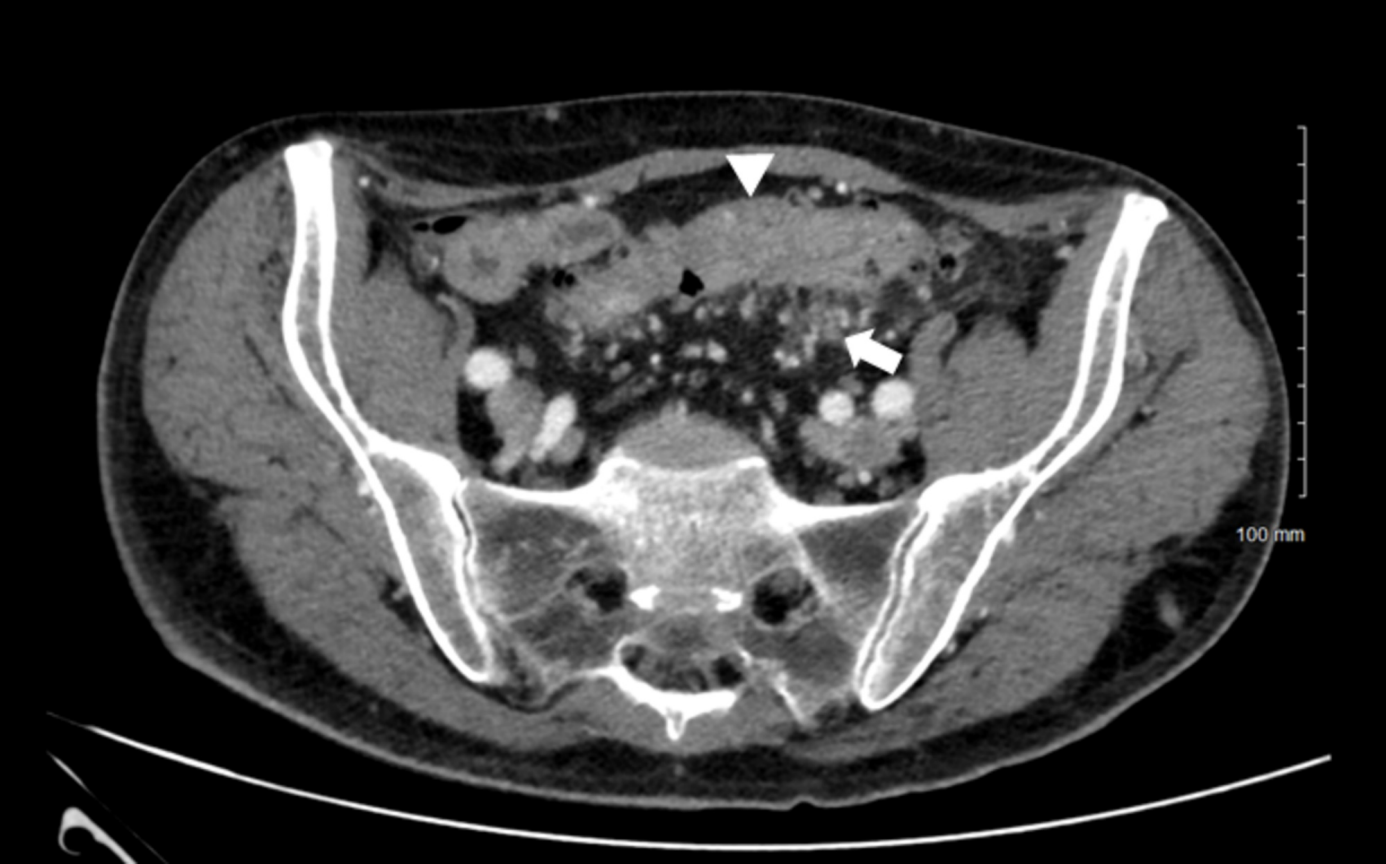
CT of the abdomen obtained two days prior to admission shows wall thickening and perisigmoid fat stranding. Wall thickening is marked with an arrow head and the perisigmoid fat stranding is marked with an arrow.
